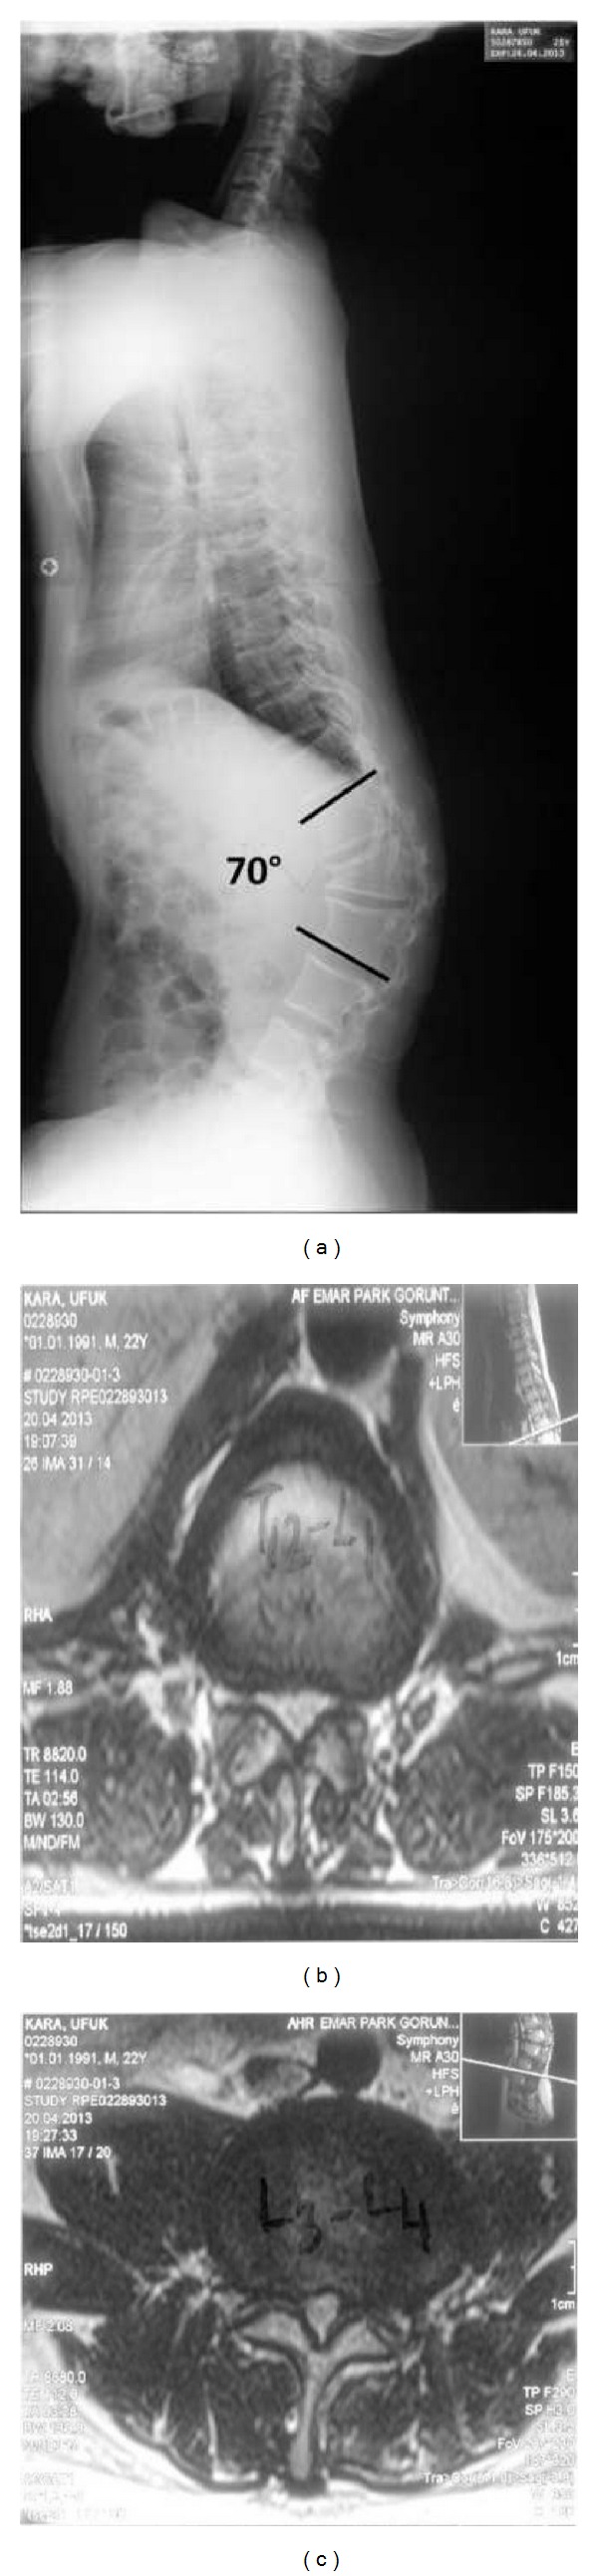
(a) Full spine X-ray showed an angular kyphosis with 70 degrees at the thoracolumbar area; (b)-(c) MRI studies showed a significant stenosis between levels T11 and L5 (MRI: magnetic resonance imaging).
